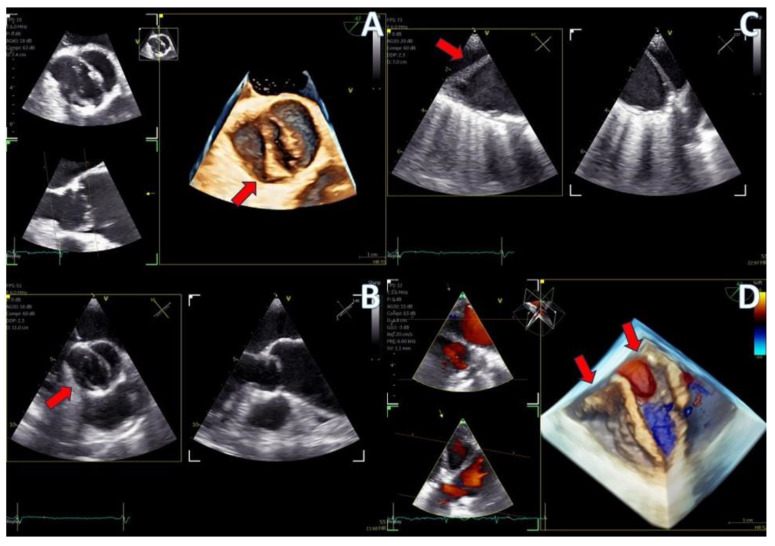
(A) A 4D demonstration of a true−bicuspid aortic valve (red arrow), (B) Biplane 2D demonstration of a true−bicuspid aortic valve (red arrow), (C) Chronic dissection of descending aorta (red arrow showing the wall of the true lumen), (D) 3D volume-rendered color Doppler image showing the true (right arrow) and the false lumen (left arrow).
